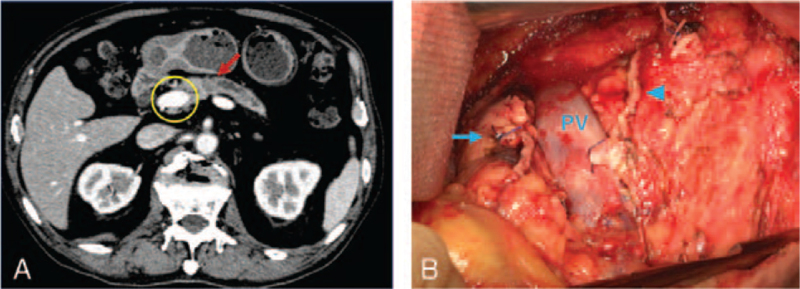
Computed tomography and intraoperative findings in case 1. (A) The image shows a 15-mm mass in the pancreatic body (red arrow), dilatation of the caudal end of the main pancreatic duct, and the portal annular pancreas (yellow circle). (B) Following pancreatic transection, the ventral (blue arrow) and dorsal (blue arrowhead) margins of the portal annular pancreas can be observed. PV = portal vein.
